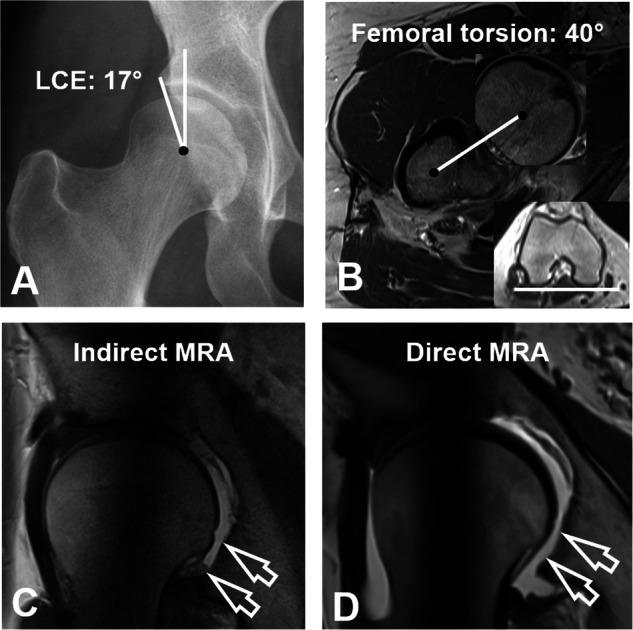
30-year-old woman with hip pain due to acetabular dysplasia and excessively high femoral torsion. A AP pelvis radiograph with a decreased lateral center-edge (LCE) angle of 17°. B, C The patient was referred to indirect MR arthrography of the hip. B Axial T1-weighted images show markedly increased femoral torsion of 40°. C Radial proton density-weighted turbo spin echo image shows postero-inferior femoral head decentration (arrows). D Two years later the patient underwent repeated imaging with direct hip MR arthrography with radial images showing femoral head decentration as well (arrows)
